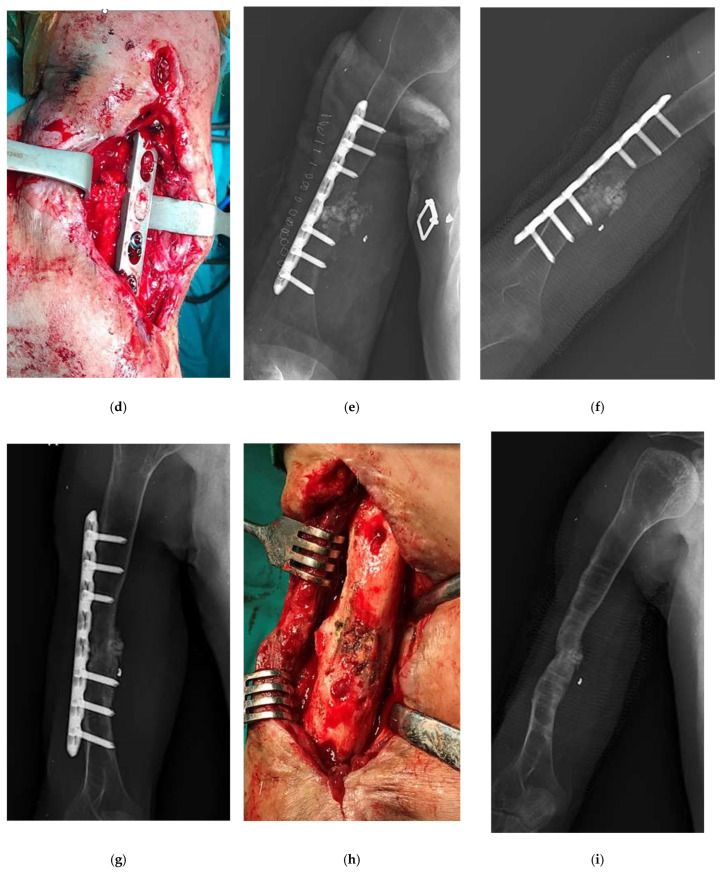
An example case of use of Silver-Doped Synthetic Bone Grafts in A 32-year-old male patient with right humerus osteomyelitis after internal fixation (a,b) AP radiograph of the right humerus before and after debridement and plate fixation. (c) The sinus tract and the extent of infection can be seen on the lateral side of the arm. (d,e) The plate and screws were not removed, cavity was packed with synthetic bone grafts. (f,g) The radiograph showed that the graft had adapted to the bone, and later there was bone continuity (h) When the implant was removed during surgery, it was visually seen that bone continuity was achieved and the graft was incorporated (i) The radiograph showed bone union and consolidation in the second year.
